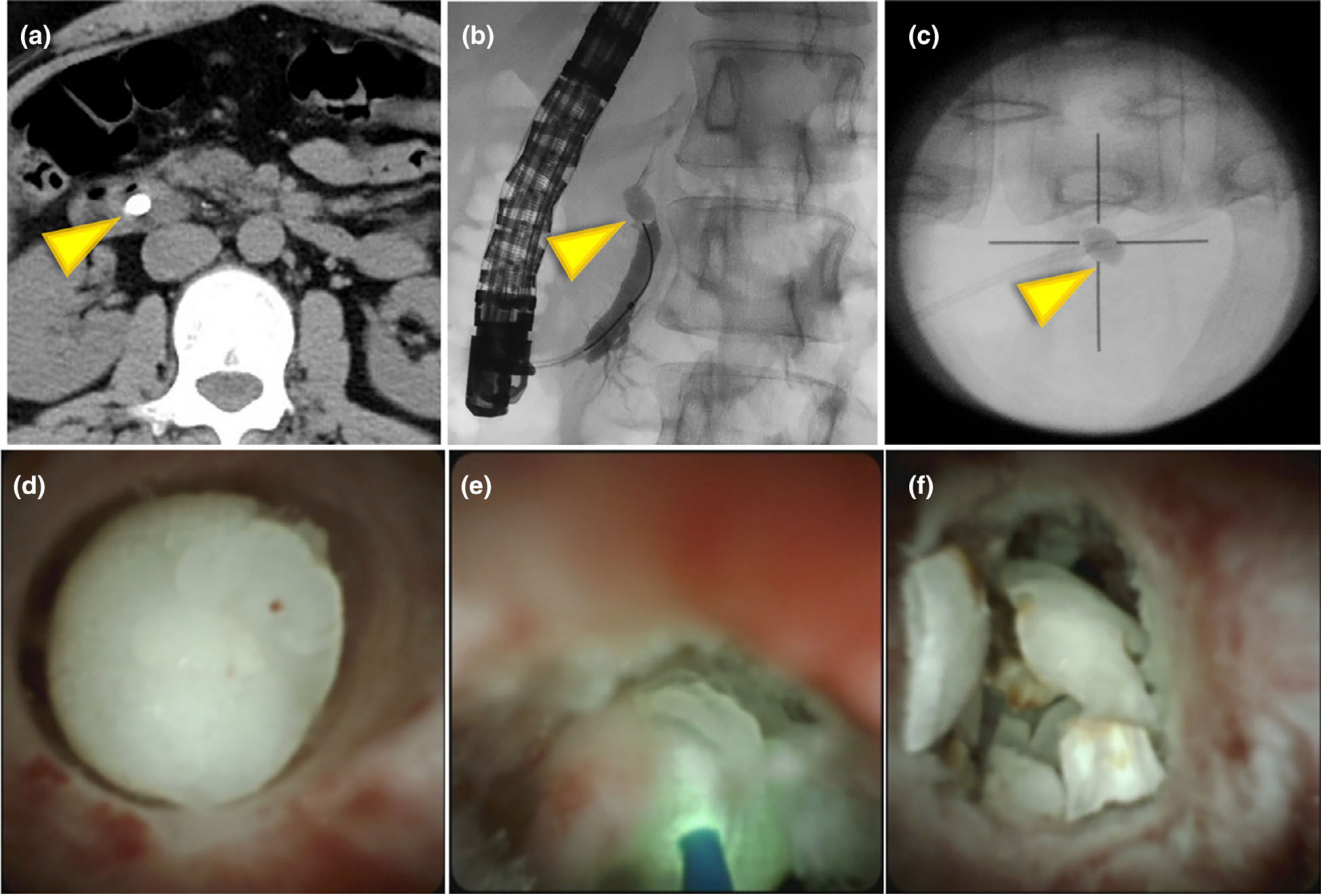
A case of refractory stone fragmented using laser lithotripsy. (a) Symptomatic 10 mm stone at the head of the main pancreatic duct (computed tomography value: 1750 Hounsfield units). (b) Endoscopic retrograde pancreatography showed impacted stone. (c) Five sessions of extracorporeal shock wave lithotripsy were performed; the procedures were not effective. (d) Use of peroral pancreatoscopy (POP) (SpyGlass DS; Boston Scientific). (e) Performing laser lithotripsy. (f) POP showing stone fragmentation.
